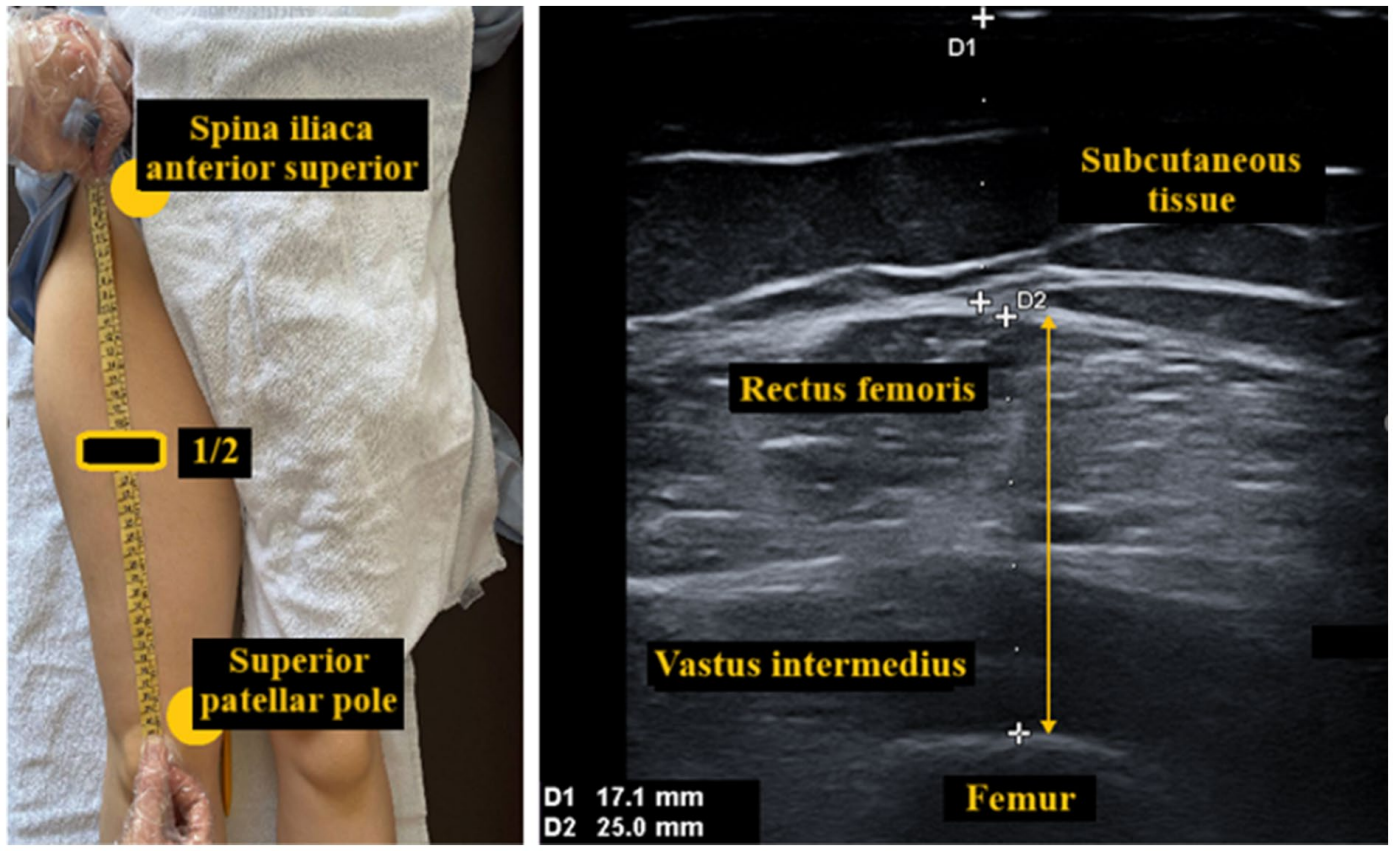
Evaluation of anterior thigh muscle thickness by ultrasonography.
